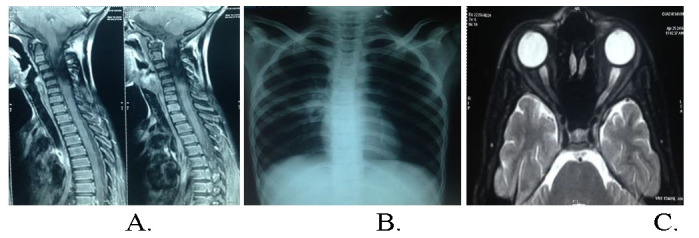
Radiological imaging of spinal cord and lung of Case 1 A. MRI of the spine suggestive of longitudinally extensive transverse myelitis; B. Chest X-Ray showing Koch’s lesions with cavitation in the right mid-zone, which was suspected to be tubercular; C. T2 Fat suppressed axial MRI of the orbits revealing hyperintensity in bilateral optic nerves
